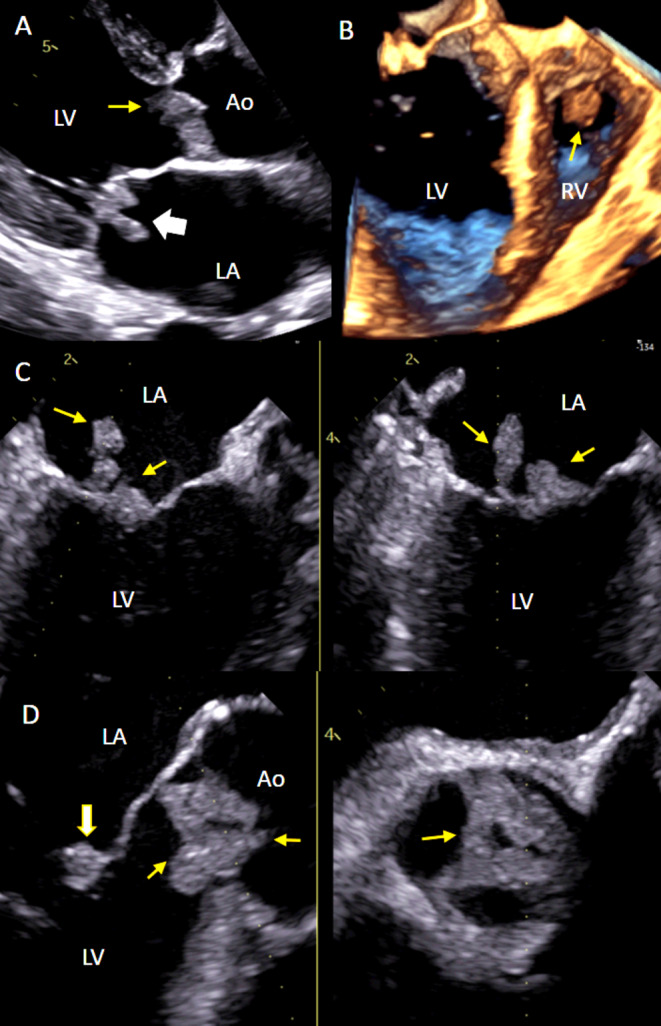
Follow‐up TTE and TEE. (A) TEE parasternal long axis view showing aortic valve masses on both cusps (yellow arrow) and mitral valve mass on both leaflets (white arrow). (B) 3D TEE showing right ventricular mass (yellow arrow). (C) TEE X‐plane image from the mid‐esophageal position, left: Long axis view, right: Mitral commissural view showing mitral valve masses on both leaflets (yellow arrows). (D) TEE X‐plane image from the mid‐esophageal position, left: AV long axis view showing cardiac masses on all cusps of aortic valve (yellow arrow) and mitral valve mass on the anterior leaflet (white arrow), right: AV short axis view showing aortic valve masses (yellow arrow). Ao: Aorta, LA: Left atria, LV: Left ventricle, RV right ventricle.
